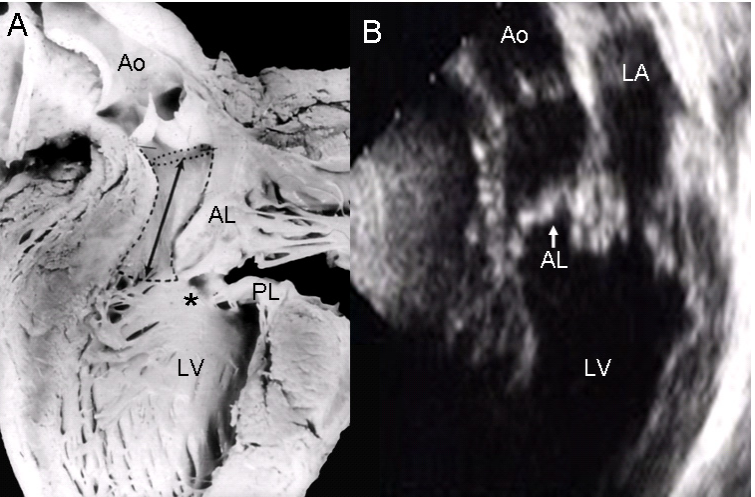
Atrioventricular septal defect with two valve orifices. (A) Internal view of the left ventricle showing obstruction of the outflow tract by invasion of the left anterior leaflet of the left atrioventricular valve (dotted lines and double headed arrow). The asterisk indicates the commissure between the septal anterior and posterior leaflets. (B) The parasternal long axis echocardiographic image shows similar features (arrow). Abbreviations: PL: Posterior leaflet. Other abbreviations as before.
